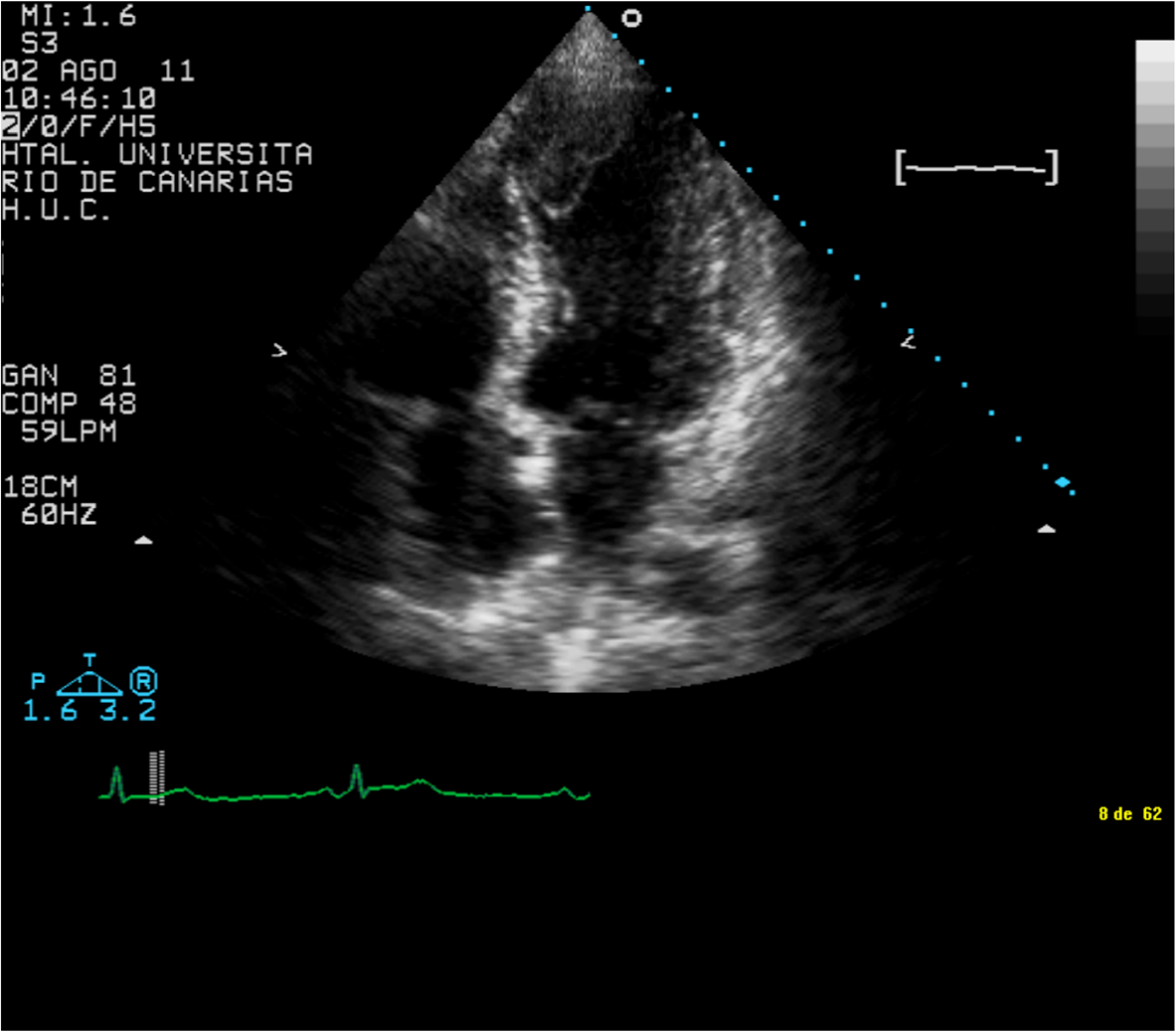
Early after admission, transthoracic echocardiography showed left ventricular inferior hypokinesis, anterior and apical akinesis, and a large intraventricular thrombus adherent to the septoapical and infero-apical endocardium.
